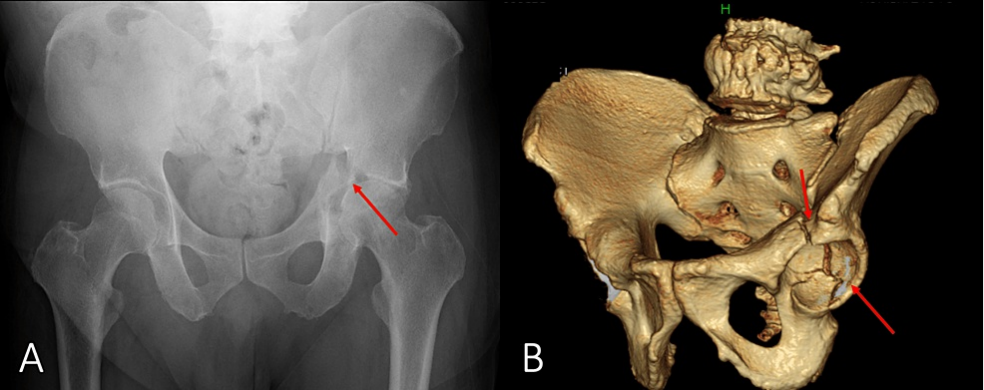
Plain radiograph and 3-D CT of the left hip obtained at the patient's primary care visit A) Frontal radiograph; B) 3-D CT of the left hip A displaced acetabular fracture is revealed (red arrows)
